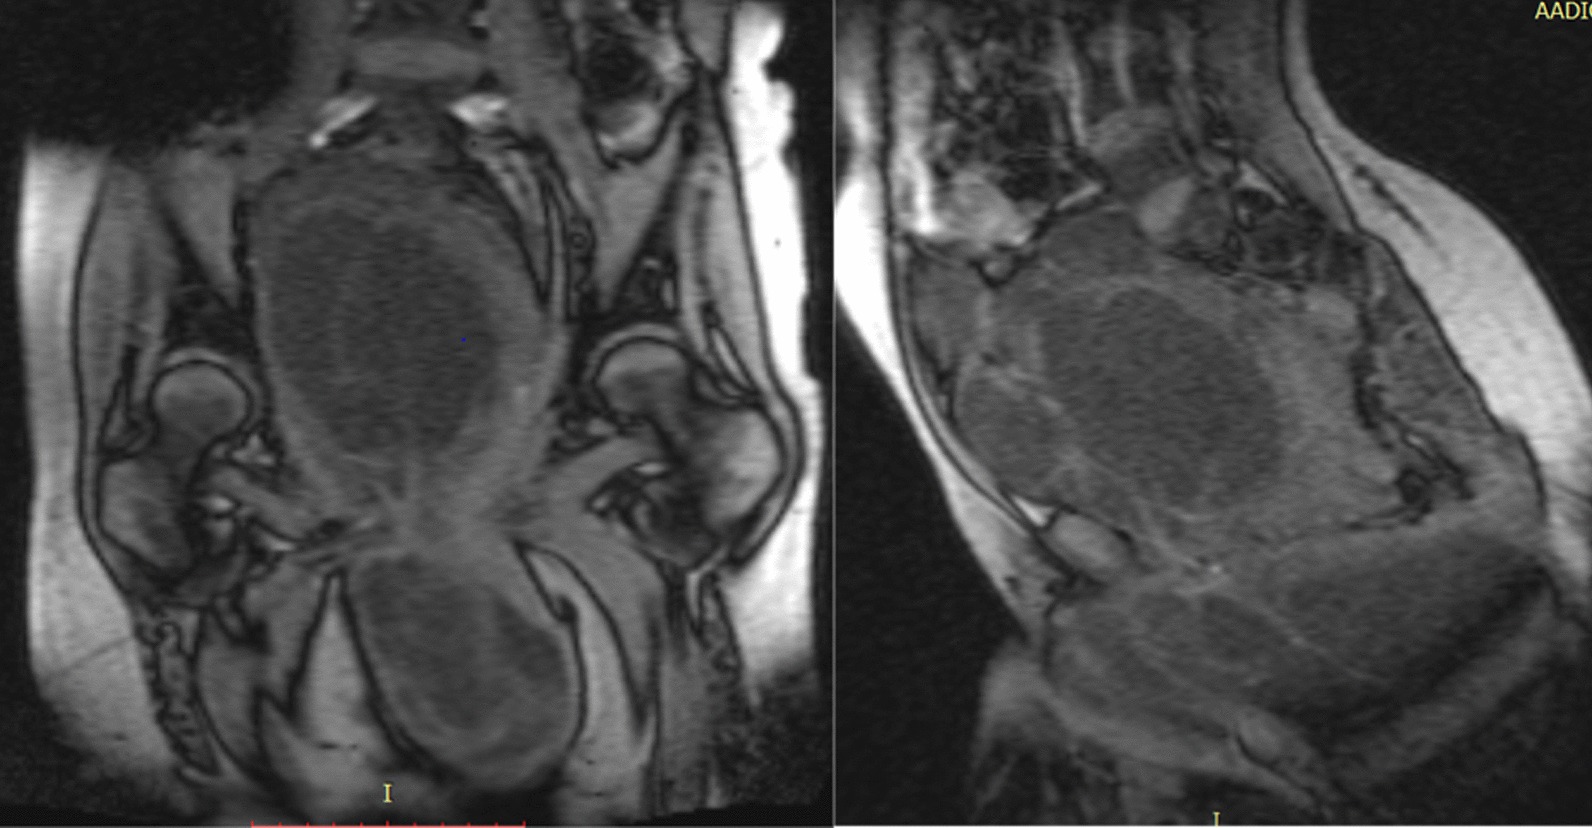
Abdomino-pelvic MRI showing the mass extending upward in to the abdominal cavity and displacing other pelvic structures
